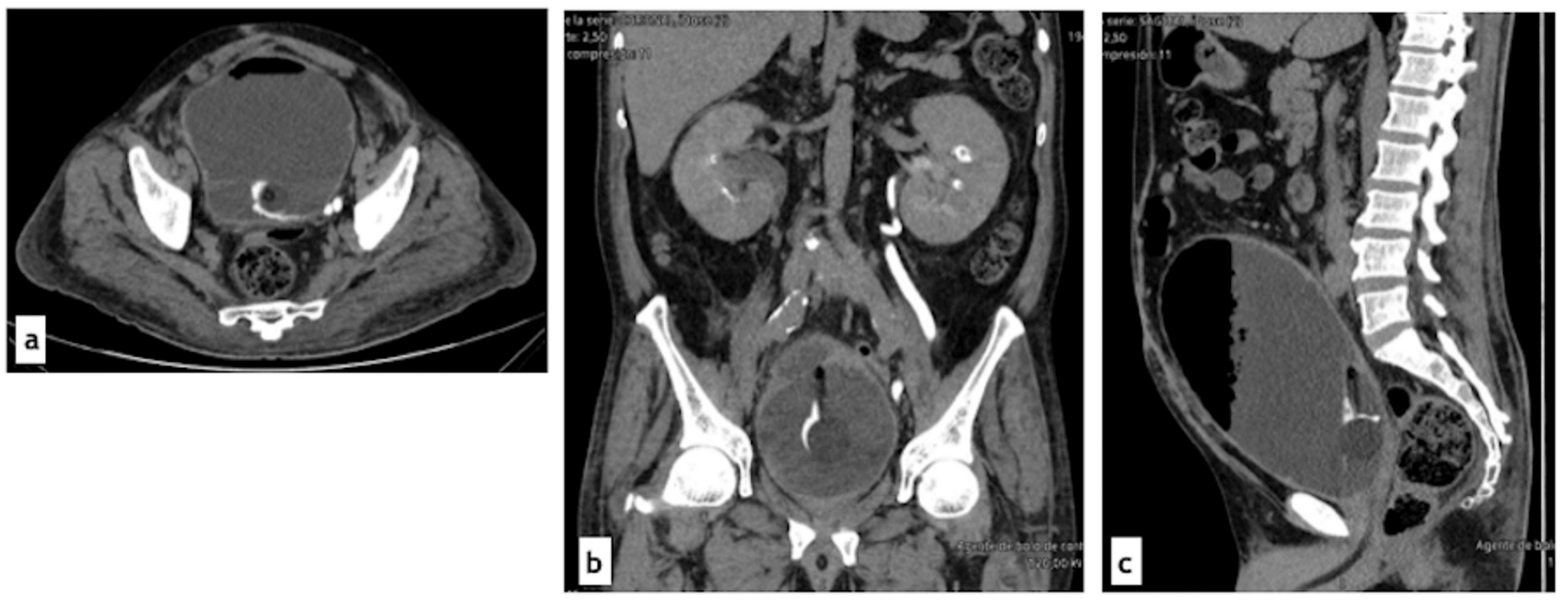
Follow-up abdominal computed tomography scan. Contrast-enhanced abdominal computed tomography, excretory phase: (a) Axial view showing persistence of intravesical sediment and gas; contrast medium delineates a crescent-shaped rim around the Foley catheter balloon without mixing with the sediment. (b) Coronal view demonstrating persistent bilateral ureteral dilation and bladder sediment non-enhancing with contrast medium. (c) Sagittal view revealing persistent bladder distention, urinary sediment, and intravesical gas.
